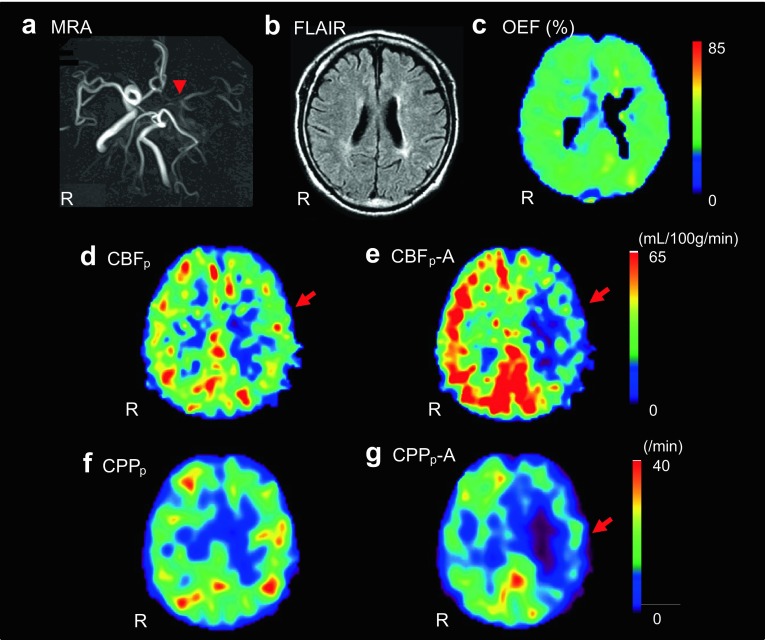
A representative case from this study. The patient had left ICA occlusion on MRA (a). *Arrowhead* shows the occlusion of ICA. MRI FLAIR image showed no significant ischemic region in the MCA territory (b). Although OEF image showed no significant increase in the left cerebral hemisphere (c), baseline CBF_p_ showed a tendency of decrease in the MCA territory (d). ACZ challenge test showed significant decrease in CBF_p_ (e) and CPP_p_ (f baseline, g after ACZ) in the affected region. The study indicated significant hemodynamic impairment because of the significant decrease in CVR and CPP, and the patient underwent EC–IC bypass surgery. CBF_p_-A and CPP_p_-A means pixel-by-pixel calculated CBF and CPP after ACZ. R on *each panel* represents *right side* of the patient. *Red arrows* show affected side of MCA territory
